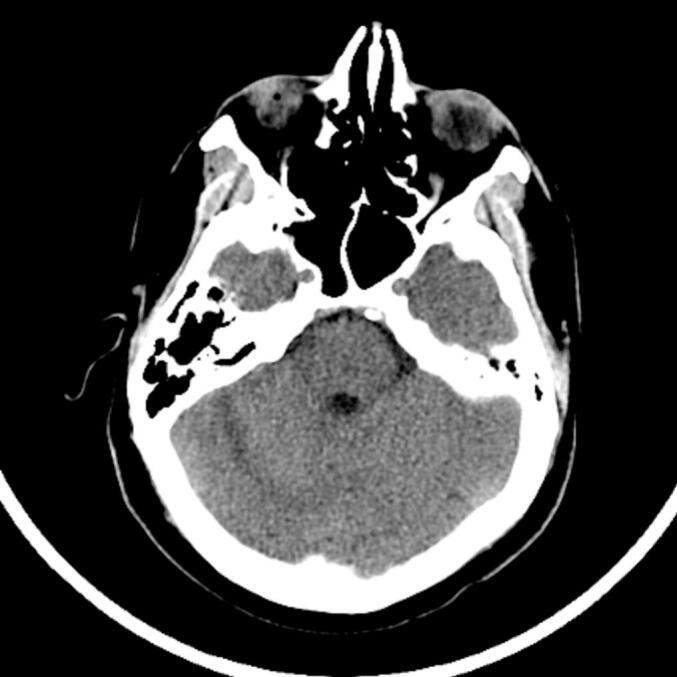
Head CT without IV contrast 1 month postoperatively demonstrating complete resolution of cerebellar hemorrhage.
